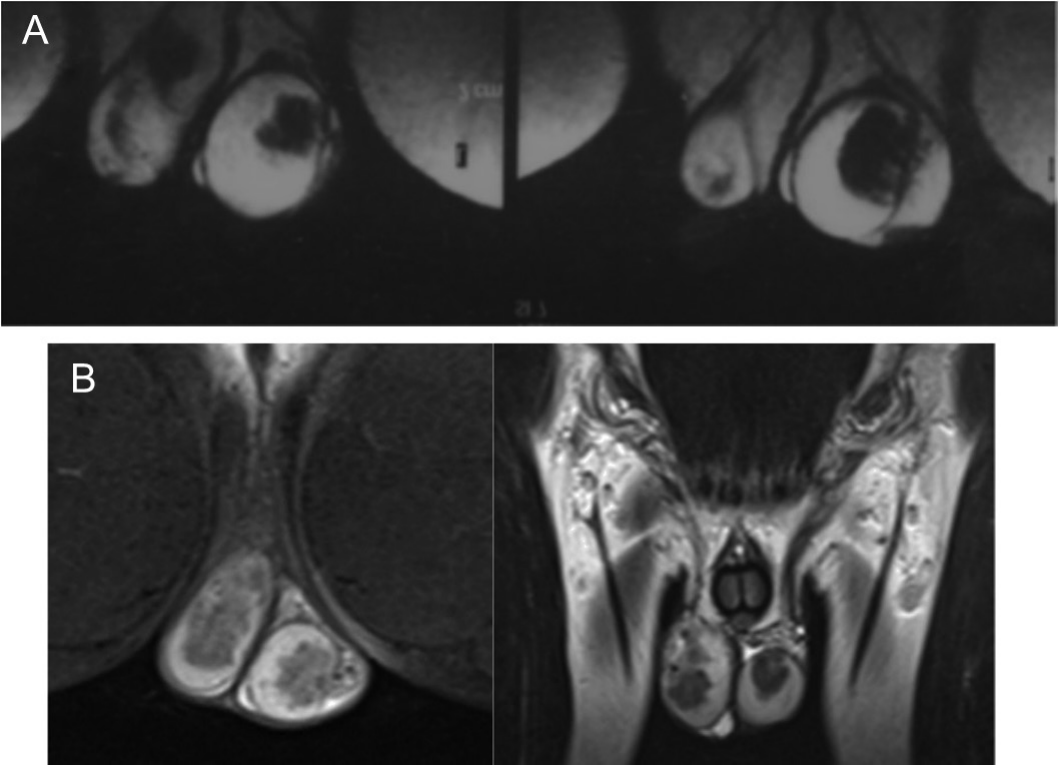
Testicular MRI in patients with TART. (A) Patient 1 during the follow-up, TART is spread around the testicular hilus. (B) Patient 3 with a large TART involving the entire testis. This patient underwent orchiectomy due to the significant discomfort.
